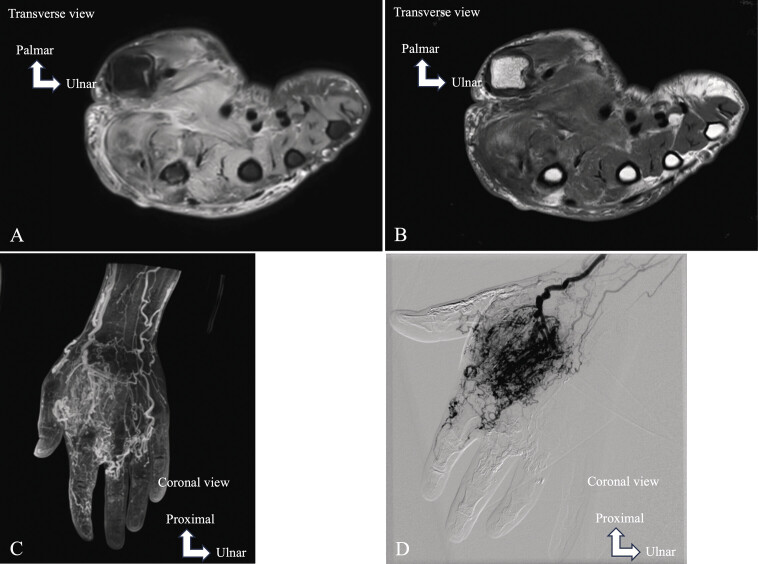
Chronic myositis due to an arteriovenous malformation of the thenar eminence and first commissure. MRI on axial PD FS (
